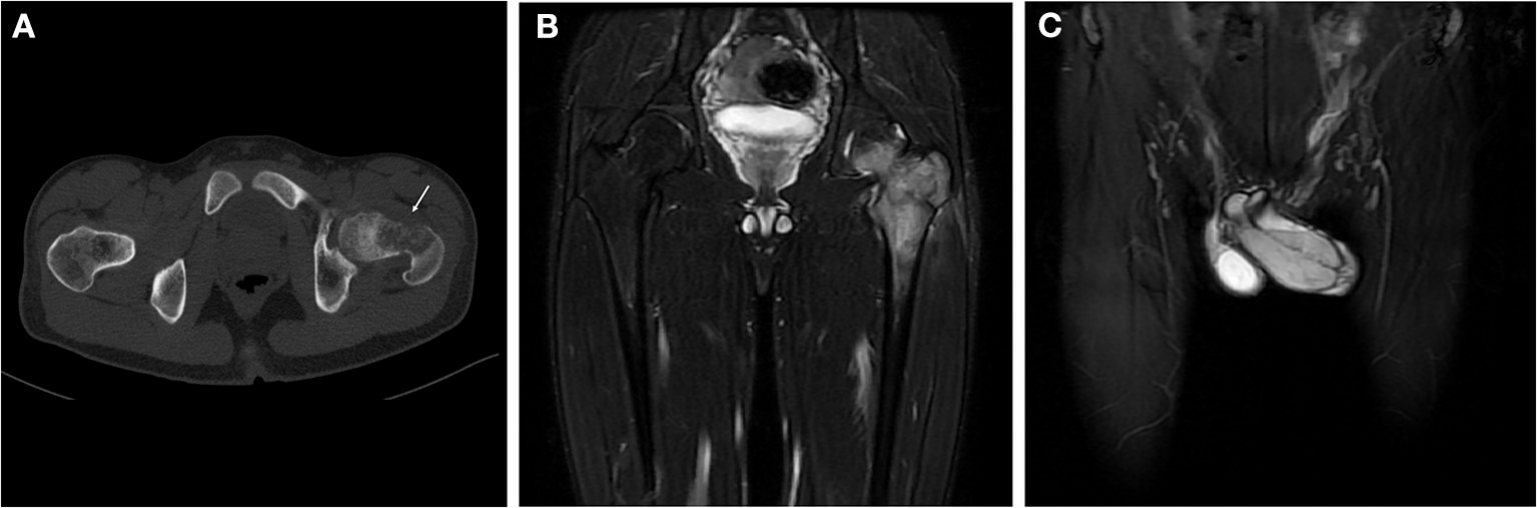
Radiological images of the pelvis. Axial CT (A, bone window) showed an aggressive lytic lesion involving the left femoral neck and trochanteric region associated with cortical destruction and limited periosteal reaction. A coronal fat-saturated T2-weighted MRI image of the pelvis (B) demonstrated a heterogeneously high signal lesion in the left proximal femur that was limited in the medullary cavity without extraosseous extension. Meanwhile, MRI images (C) showed a hyperintense soft tissue mass in the dorsal side of the penis with poorly defined zones of high signal in the *corpus cavernosum* around the tumor.
